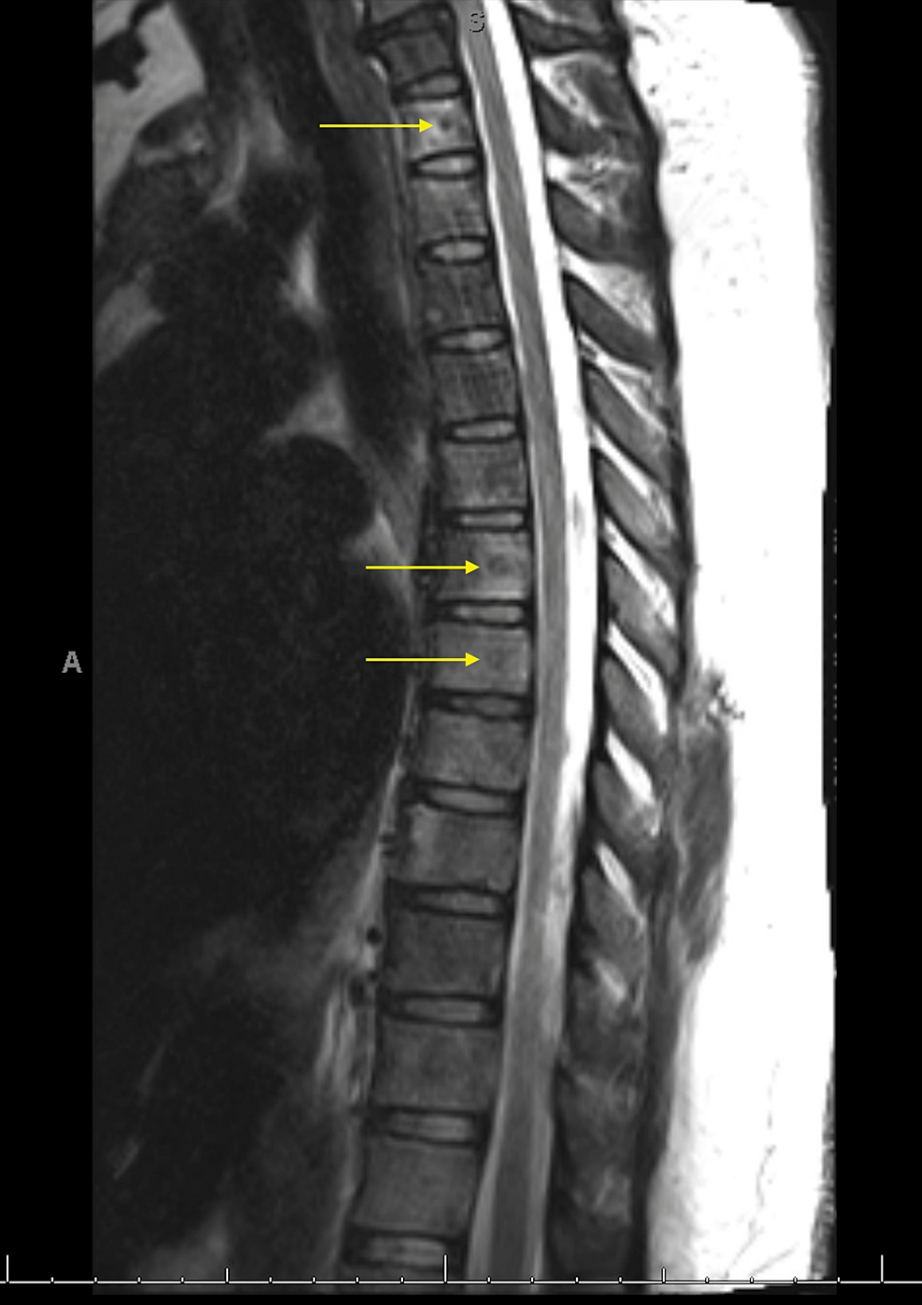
MRI of the spine MRI of the spine revealed multilevel metastatic disease in the thoracic spine.
